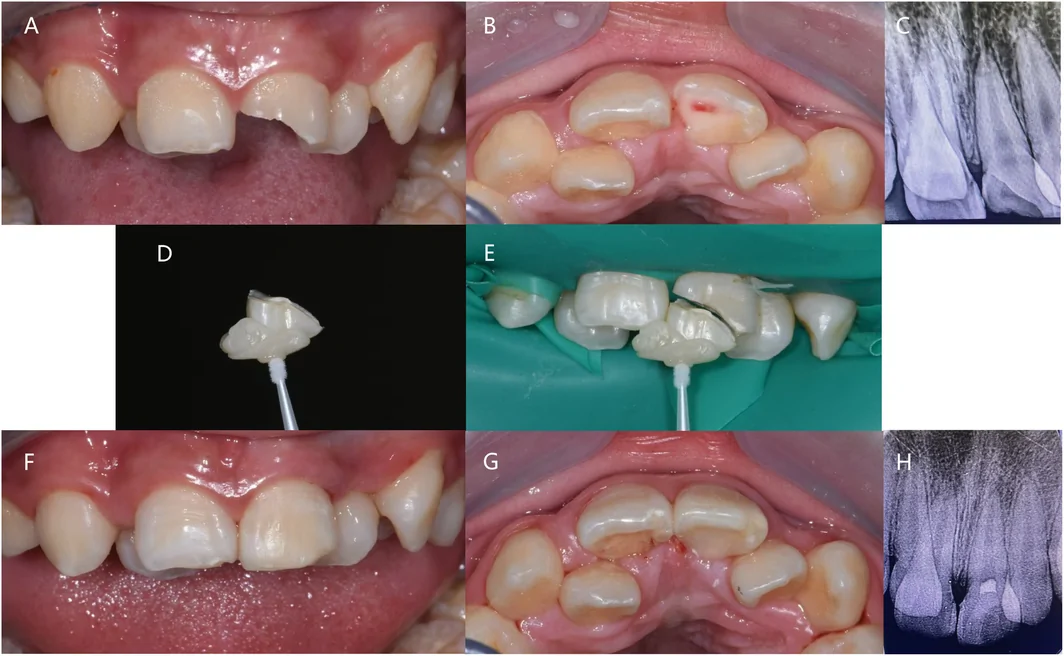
Clinical presentation of an 11‐year‐old male patient with traumatized maxillary anterior teeth; the fractured crown fragment was preserved in milk for 7 h post‐injury. (A, B) Intraoral clinical photographs reveal crowded maxillary anterior dentition. Tooth 11 exhibited mesial incisal enamel loss without pathological mobility. Tooth 21 sustained an oblique crown fracture involving one‐third of the clinical crown, accompanied by a palatal fracture margin extending approximately 0.5 mm subgingivally and pulp exposure. The pulp exposure measured 1 mm in diameter, and the fractured fragment demonstrated a distal incisal defect when anatomically repositioned. (C) Preoperative periapical radiographs. Tooth 11 presented Nolla stage 10 root development, continuous root canal walls, and widened periodontal ligament space. Tooth 21 showed a pulp‐chamber‐involving crown fracture, intact root canal walls, and Nolla stage 10 root maturation with widening of the periodontal ligament space. (D) Following minimally invasive partial gingivectomy with an electrosurgical unit, a custom resin wing was fabricated on the isolated fractured crown fragment. (E) Rubber dam isolation was implemented, and passive seating of the fractured crown with resin wing support was verified intraorally. (F, G) After completion of partial pulpotomy on tooth 21, the fractured crown fragment was passively positioned and bonded with resin wing assistance. A shallow concave bevel was prepared along the labial fracture margin, followed by resin reinforcement, occlusal adjustment, and finishing/polishing of the restoration. (H) Postoperative periapical radiograph displays radiopaque pulp‐capping material at the root canal orifice of tooth 21; the reattached crown fragment remained undisplaced with a uniformly thick adhesive interface.
